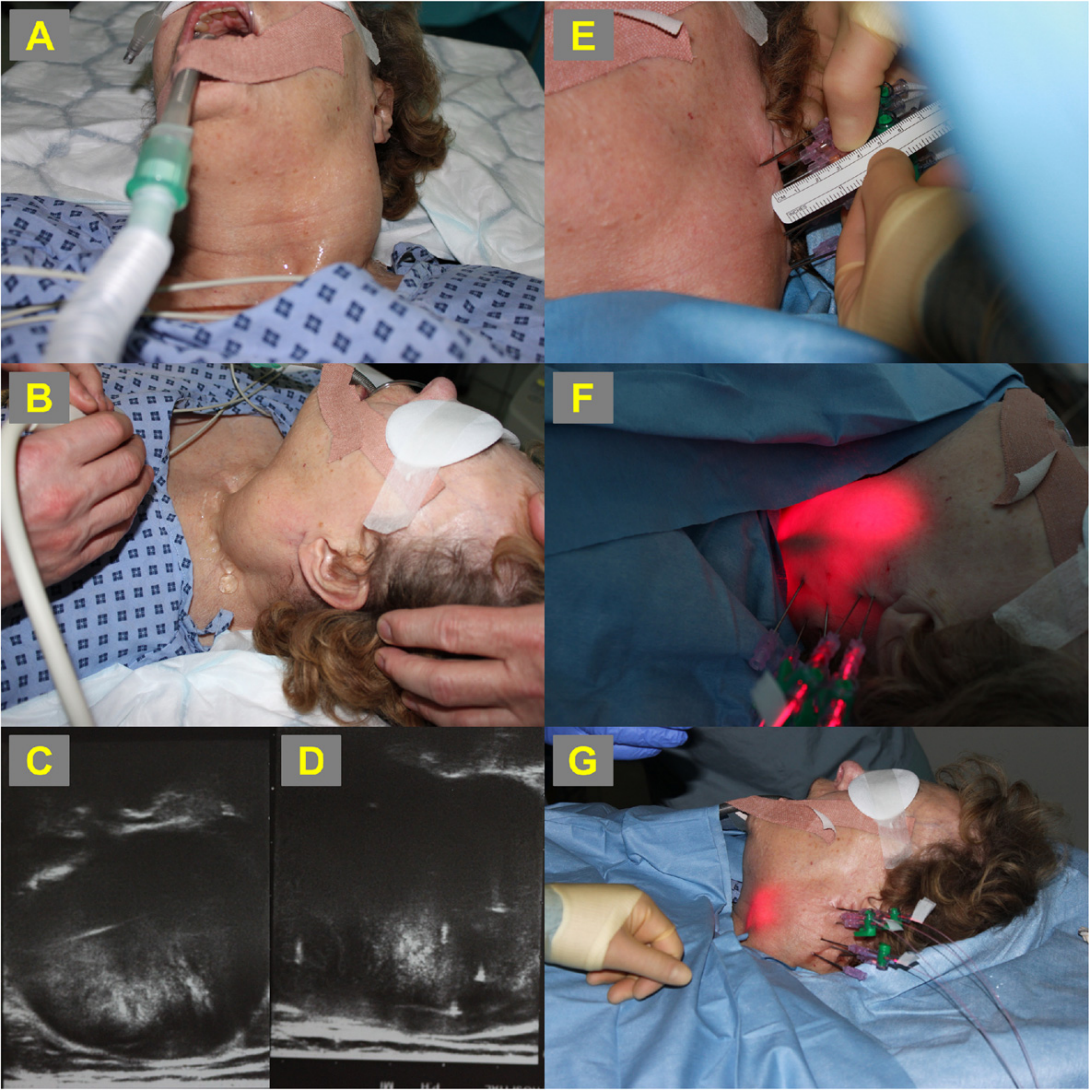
PDT of neurofibroma. A: Intra-operative image showing the large mass of the left neck. B: Intra-operative ultrasonography assessing the centre and periphery of the lesion as well as any neurovascular involvement or involvement of hollow organs. C and D: On-table US images showing the neurofibroma and its boundaries. E: Needle insertion into lesion prior to fibre insertion. F and G: light delivery to initiate the photochemical reaction.
